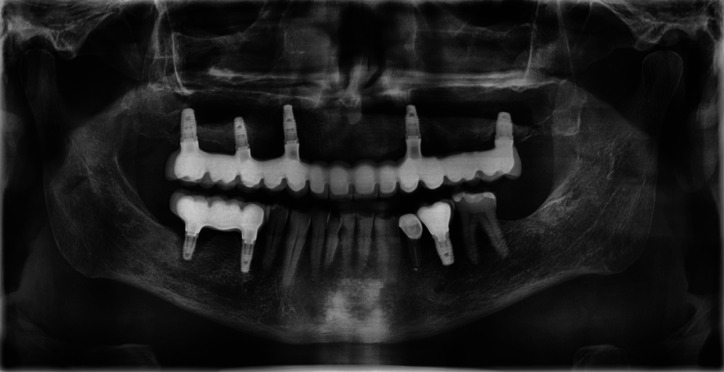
Orthopantomography carried out after one year of follow-up in which the patient showed no symptoms of sinus infection.
